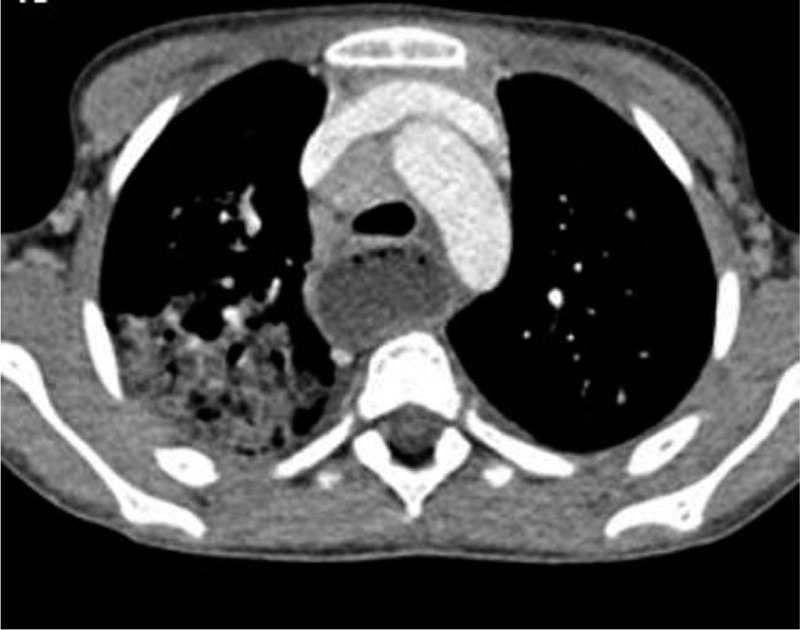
Chest computed tomography scan showing right parenchymal lobar pneumonia associated with air bronchogram, diffuse “ground glass” images, multiple mediastinal enlarged lymph nodes, and a dilated esophagus filled with solid material.
